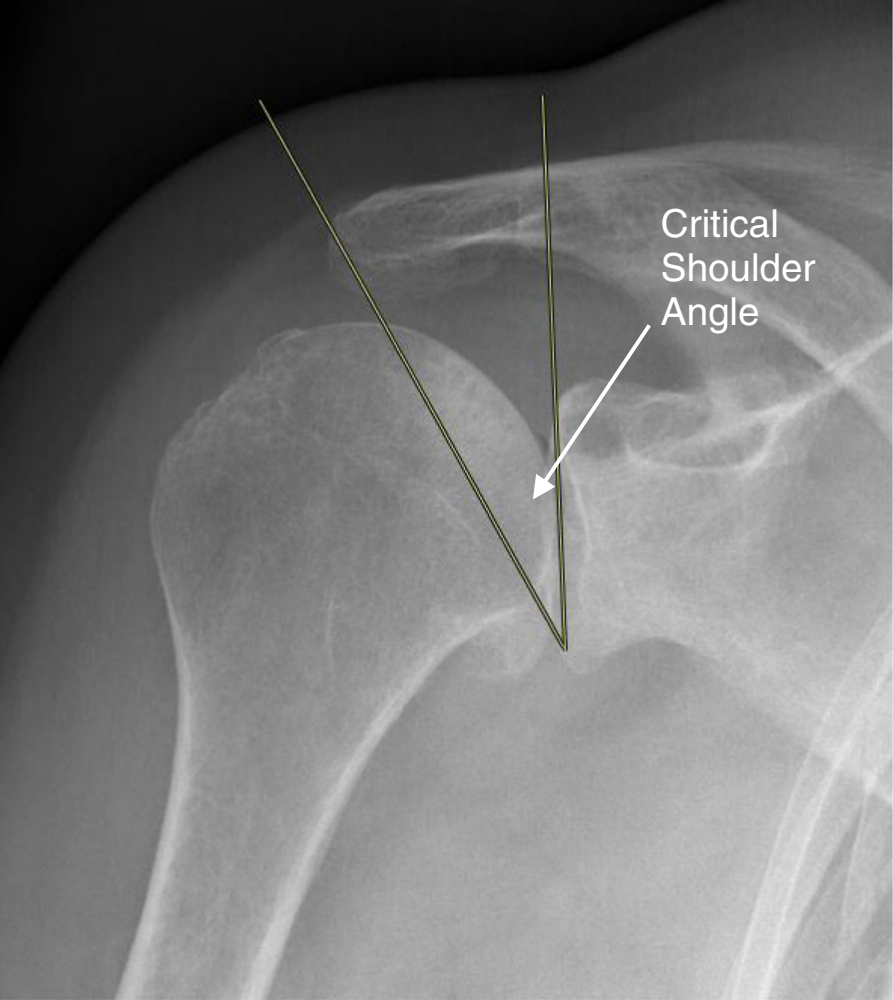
Radiograph of a right shoulder showing the assessment of the critical shoulder angle
